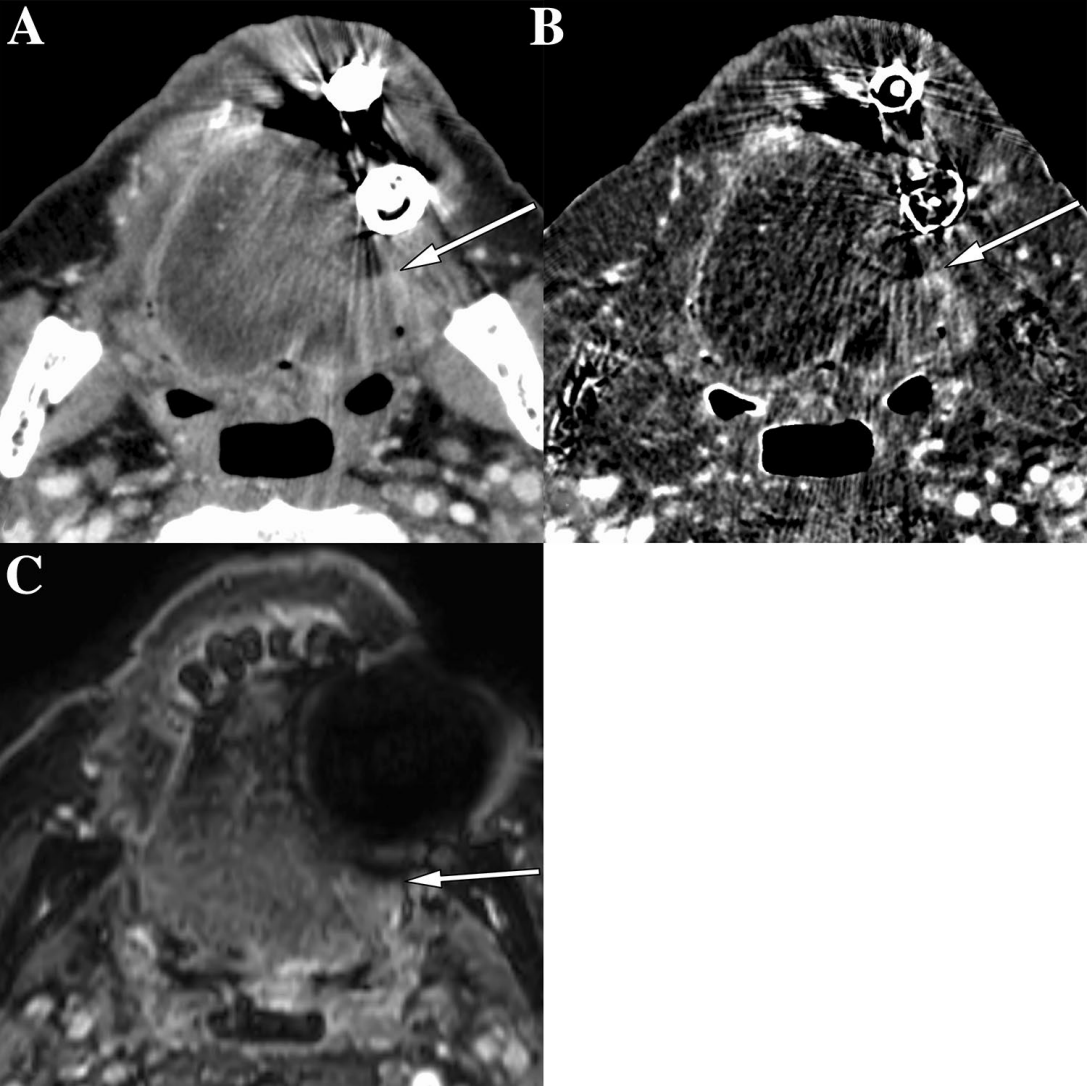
Images of a 74-year-old man with squamous cell carcinoma of the left border of the tongue. A, B On CT with the single-energy projection-based metallic artifact reduction algorithm (A) and subtraction iodine imaging (B), the anterior part of the tumor is delineated (arrows in A and B), and the tumor size is measurable. C On contrast-enhanced T1-weighted MR images with fat suppression, the anterior part of a tumor cannot be delineated due to dental artifacts, and the tumor size is unmeasurable
